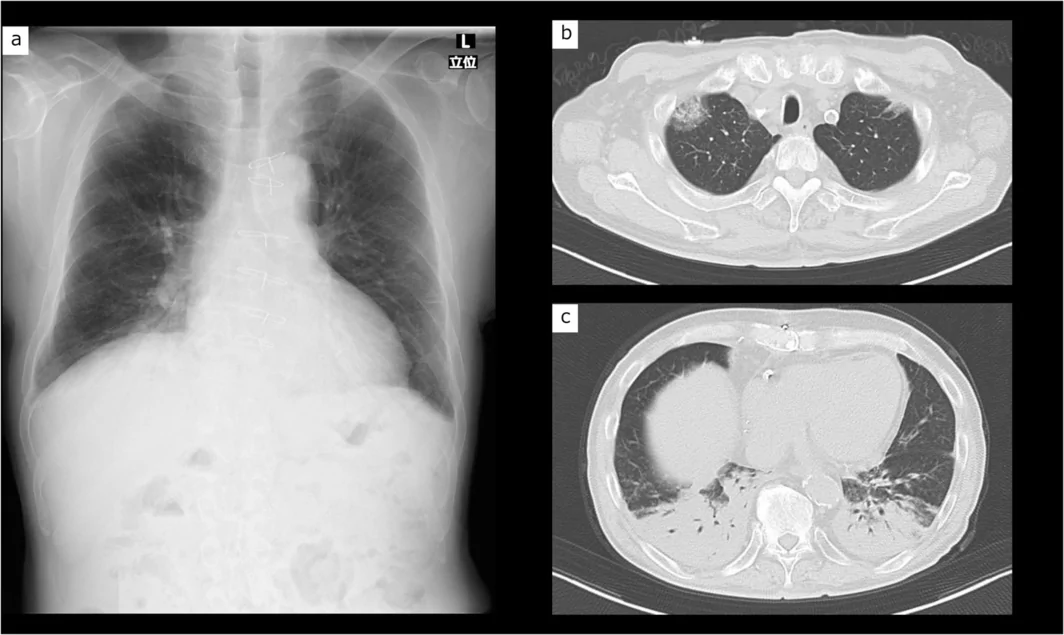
Imaging findings on admission. (a) Chest radiography and (b, c) computed tomography (CT) showing consolidations in the right upper lobe and bilateral lower lobes.
